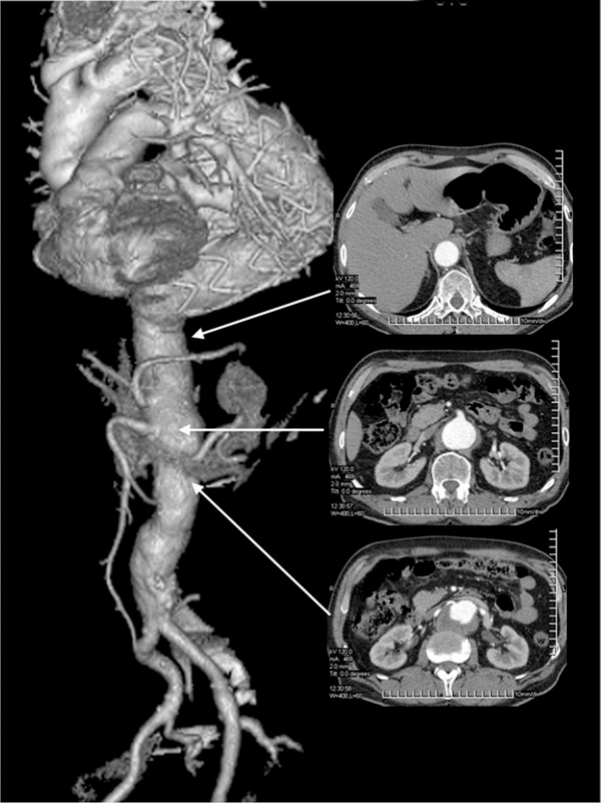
Angio CT scan of the thoracoabdominal aorta, performed 18 months after endovascular repair of thoracic aorta aneurysm: transverse reconstructions at the healthy segment of the descending thoracic aorta and at the superior mesenteric and renal artery levels.
